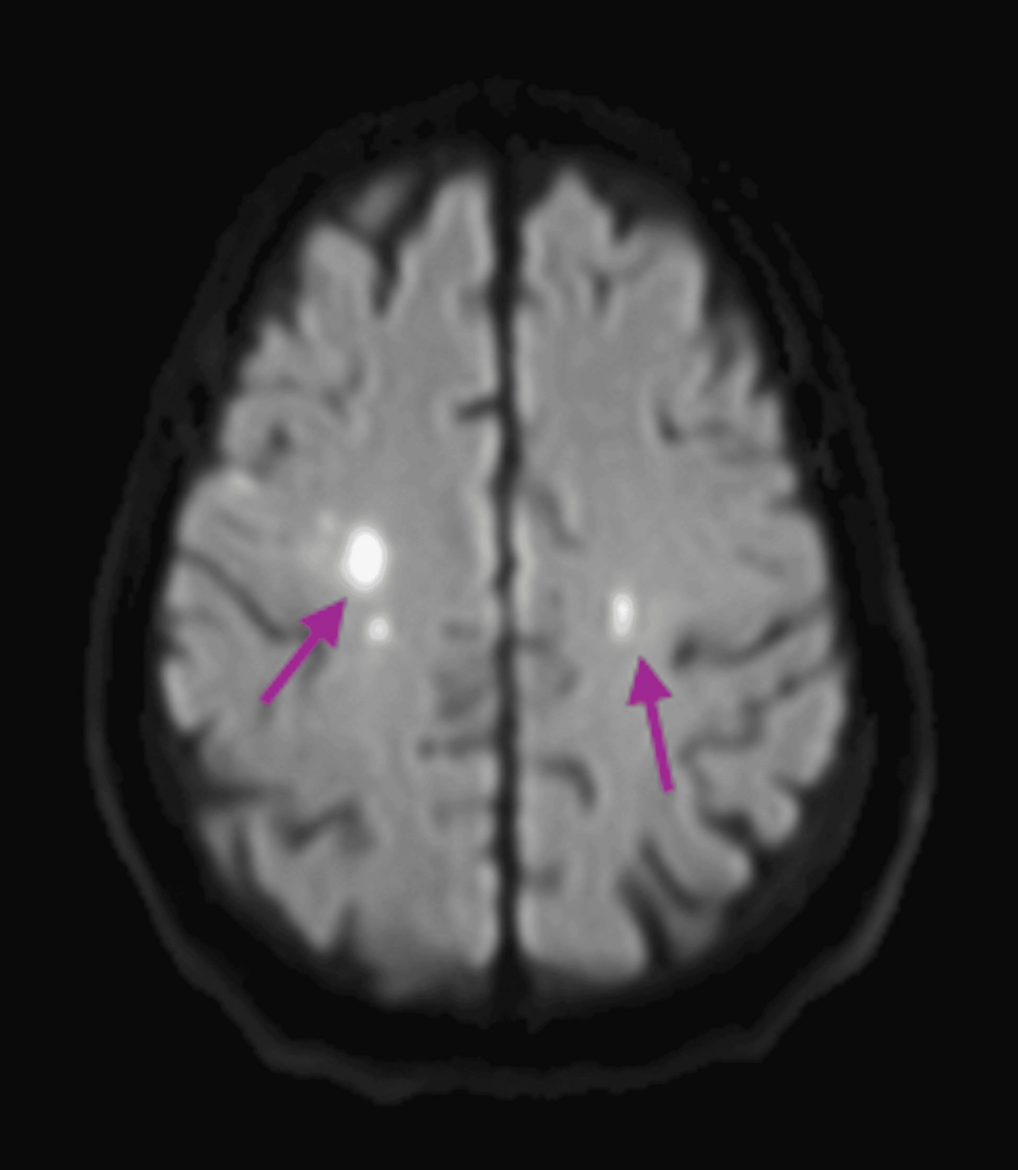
MRI of the septic emboli Axial diffusion-weighted imaging MRI sequence depicts small foci of restricted diffusion in the bilateral cerebral hemispheres in varying vascular distributions, most consistent with an embolic etiology. MRI: magnetic resonance imaging
